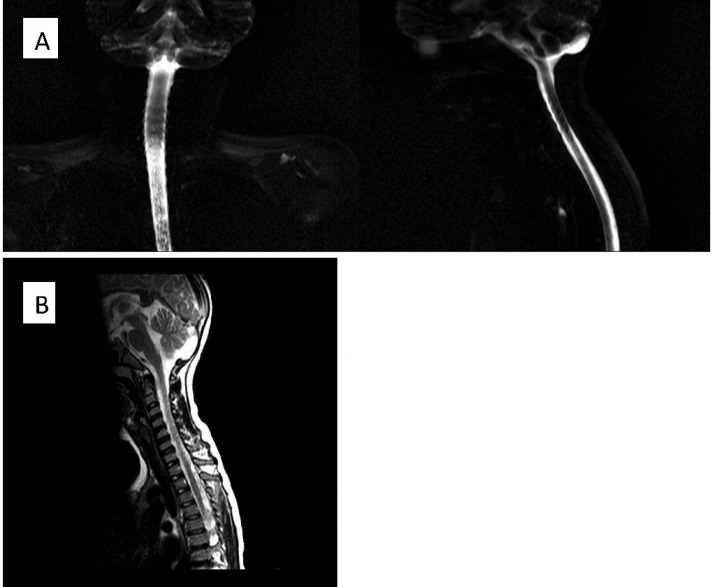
Cervical magnetic resonance imaging shows mild scoliosis and loss of cervical lordosis (A), No discopathy is notable (B).
